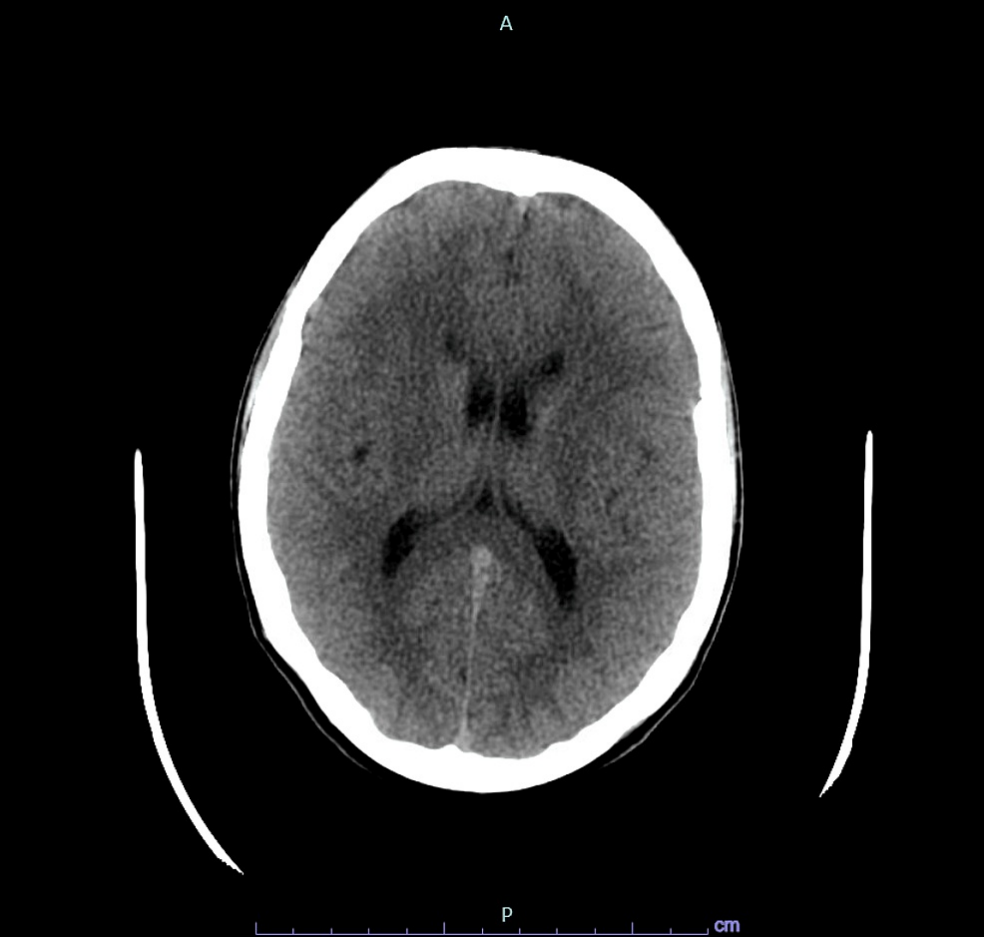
CT head without contrast showing no abnormalities CT= Computed Tomography
